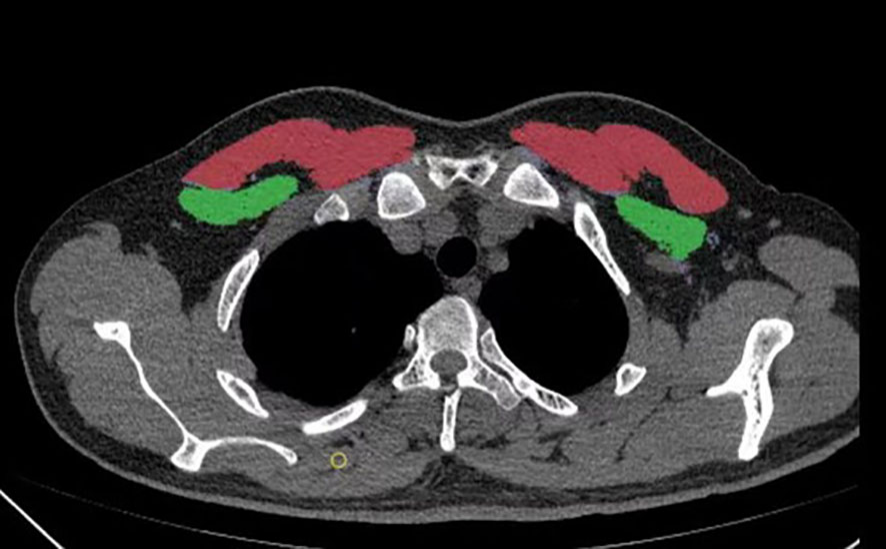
A method for determining pectoralis muscle area (PMA) by computed tomography (CT) with open-source software of 3D Slicer. a Horizontal plane of the T4 level; the pectoralis major is red, and the pectoralis minor is green.
